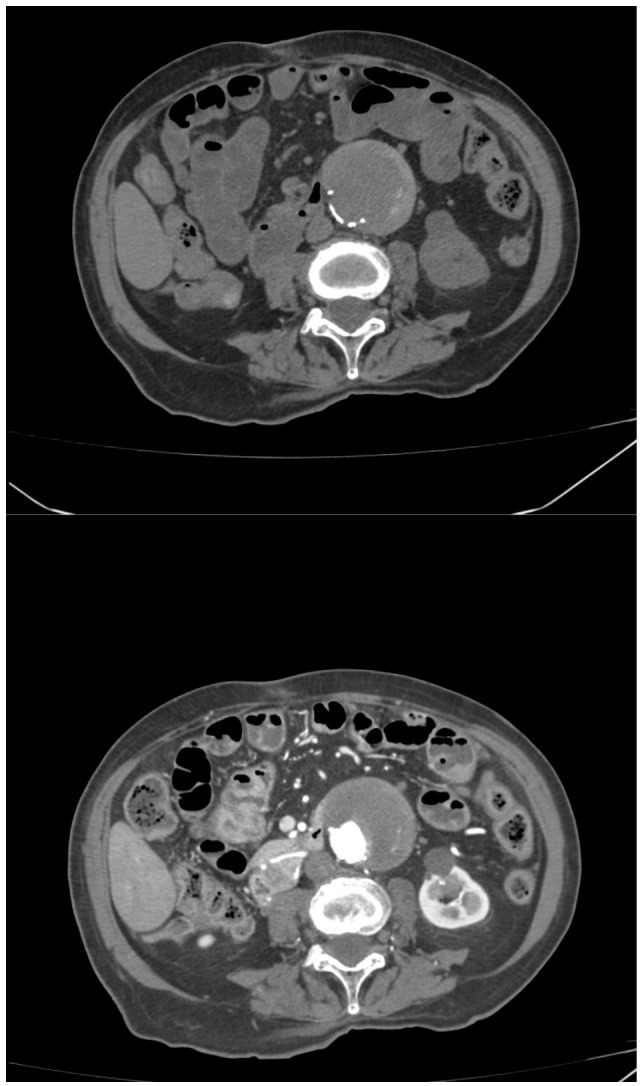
CT angiography showing a saccular infrarenal abdominal aortic aneurysm with eccentric mural thrombus and heterogeneous intraluminal components. The saccular morphology and thrombus characteristics represent imaging markers of focal wall instability and increased rupture risk.
